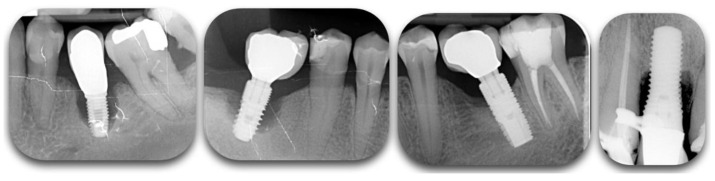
Radiographic films showing different degrees of peri-implant bone loss. The change of contrast can make the defect difficult to categorize.
